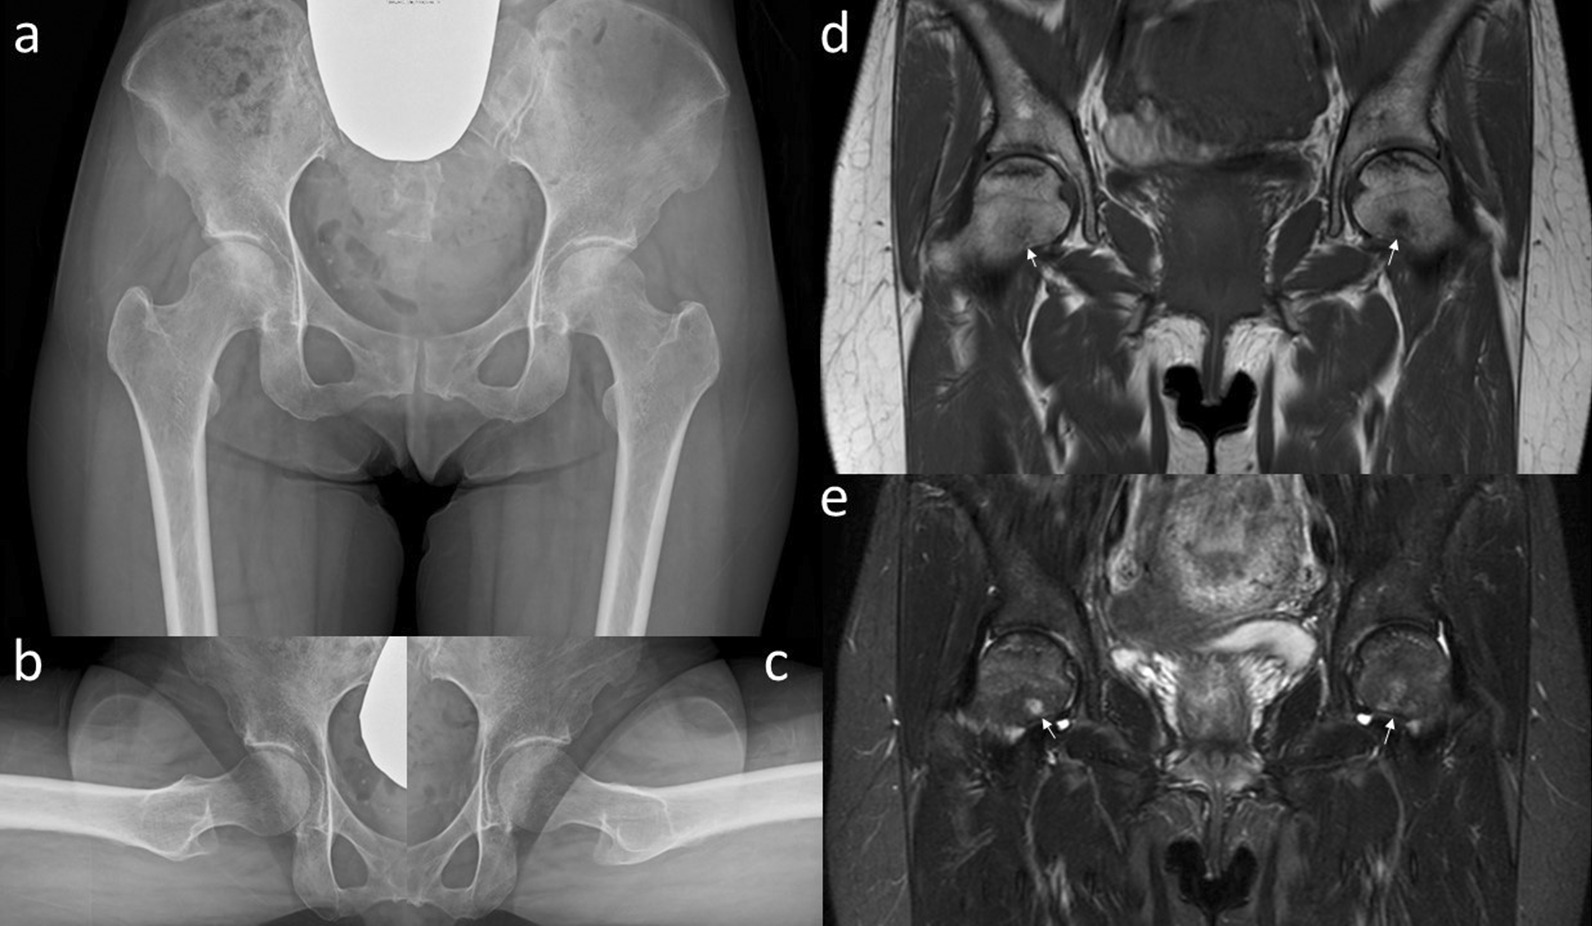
Plain radiographs a–c and magnetic resonance imaging d, e of the hip joint at 6 months after the first visit. Anteroposterior a and lateral (Sugioka) (b right hip joint; c left hip joint) views demonstrate unremarkable findings. The coronal view of a T1-weighted image of the magnetic resonance imaging d shows the presence of a low signal area in the subcapital region of the femoral head (white arrows). A high signal area appears in the subcapital region (white arrows) in the coronal view of an STIR image of the magnetic resonance imaging (e)
